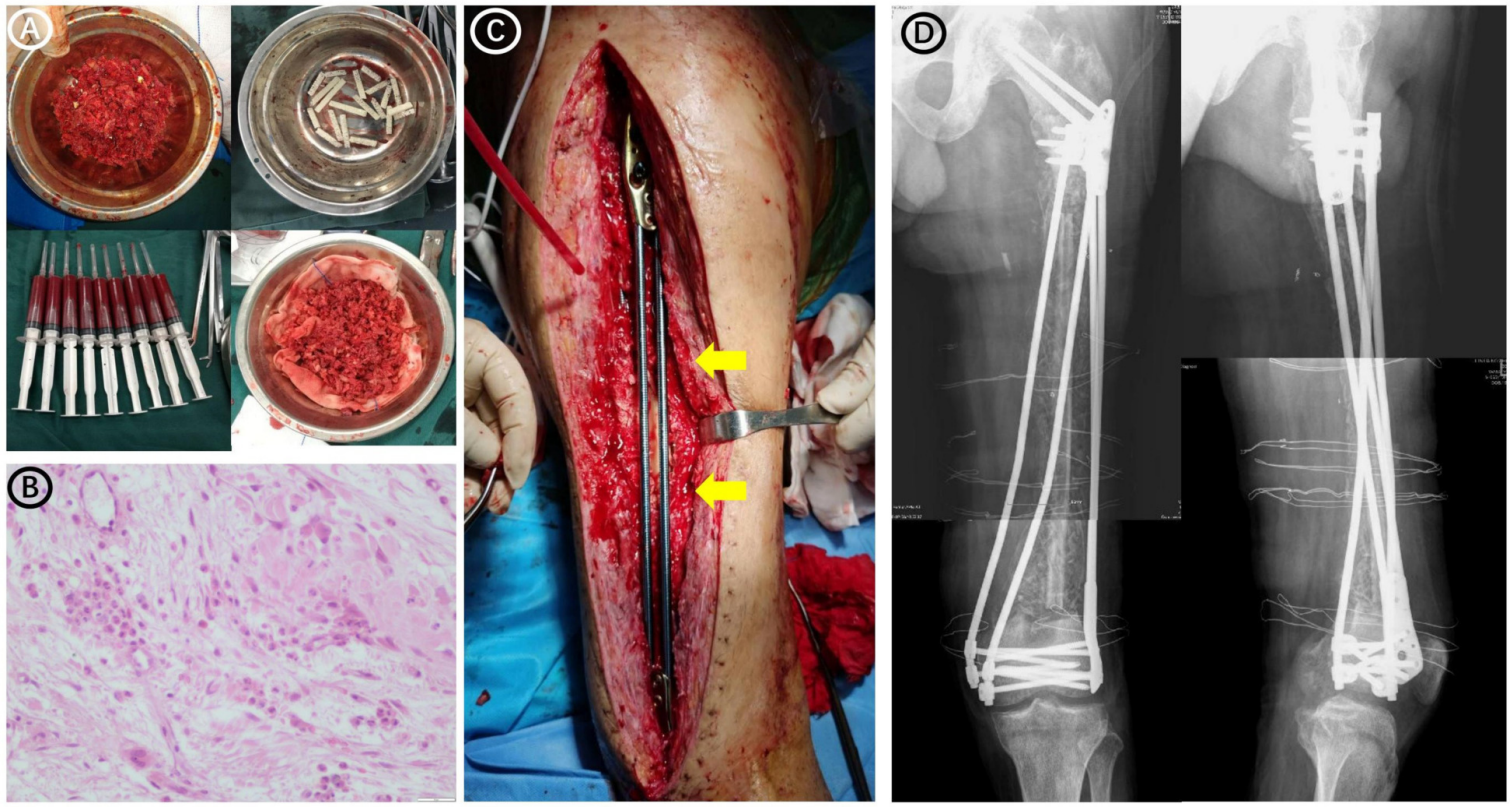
Preoperative and postoperative images of the patient undergoing a bone graft. (A) Autologous iliac bone, autologous bone marrow stem cells, and bone morphogenetic protein-loaded artificial bone used in surgery. (B) Pathological examination during bone grafting surgery revealed a few inflammatory cells infiltrating the soft tissue around the bone defect, and the number of neutrophils was less than 5 in each high-power (×400 magnification) field of view. (C) Intraoperative image showing the bridging internal fixation (yellow arrows). (D) Radiographs obtained after bone grafting and bridging of the internal fixation.
